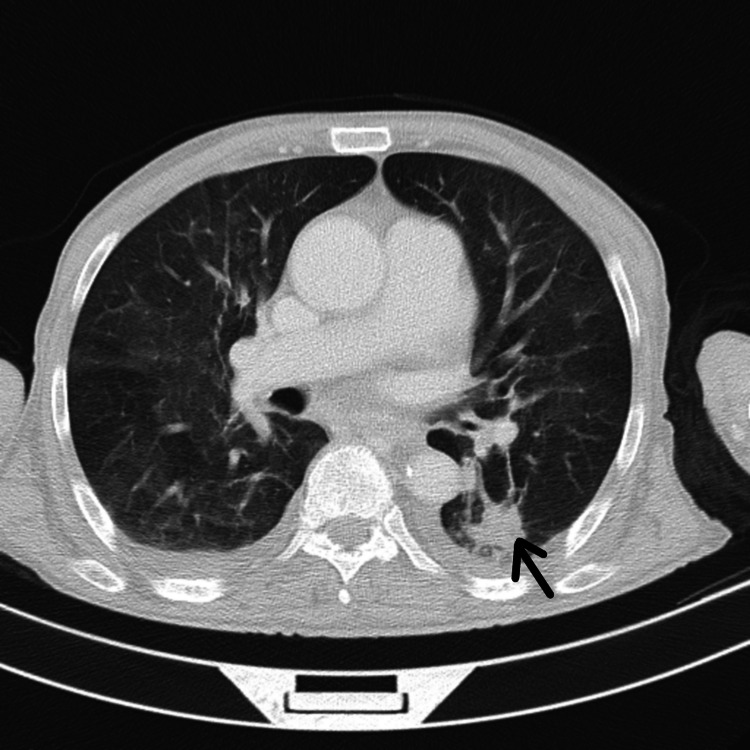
Chest CT showing consolidation with air bronchogram (arrow)
